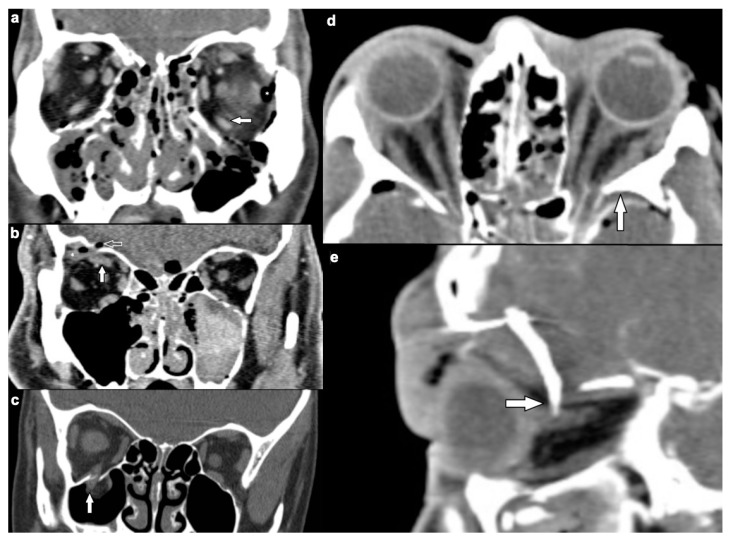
(a–c) Dislocated extraocular muscles. CE-MSCT, soft tissue window, coronal reformations. (a) Dislocation of the left inferior rectus muscle (white solid arrow) due to orbital floor fracture. Asterisk indicates orbital emphysema. (b) Displacement of the right superior rectus muscle (solid white arrow) with orbital roof fracture (white outlined arrow) and intraorbital hemorrhage (asterisk). Concomitant left hematosinus maxillaris due to midface fractures. (c) Right inferior rectus muscle (white solid arrow) incarcerated in a so-called “trapdoor fracture” of the orbital floor. (d,e) Extraocular muscles pierced by bone fragments. CE-MSCT, soft tissue window. (a) Axial reformation. Left lateral rectus muscle pierced by a fragment of the greater sphenoid wing (white arrow). (b) Sagittal reformation. Right superior rectus muscle pierced by a frontal bone fragment (white arrow), which also displaces the optic nerve (CE-MSCT = contrast-enhanced multi-slice CT).
